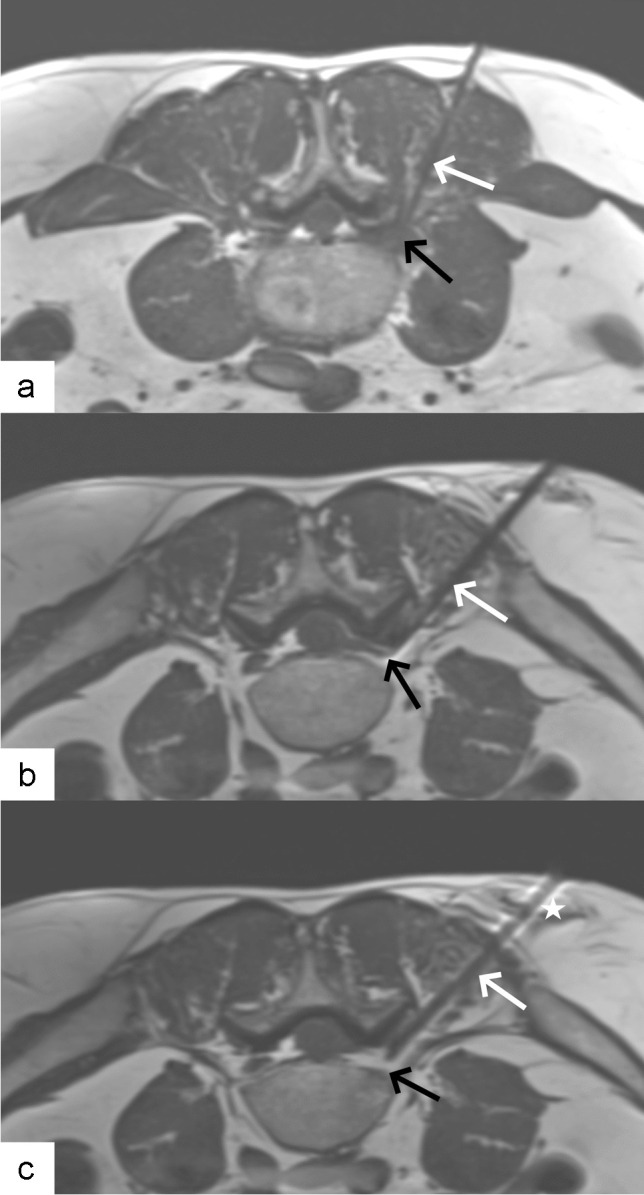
Non-contrast MRI scans obtained in nerve root infiltrations in 67-year-old man. a Axial proton density-weighted image showing 22G needle (white arrow) arriving behind fibrotic tissue (black arrow) surrounding left L4 nerve. b, c Axial proton density-weighted images showing 20G needle (white arrows) arriving behind left L5 nerve (black arrows). Hypointense needle artifact was reduced with usage of 22G needle (a) as compared with 20G needle (b and c). Note also hyperintense artifact presents on both sides of the needle in the phase-encoding direction (star in c)
